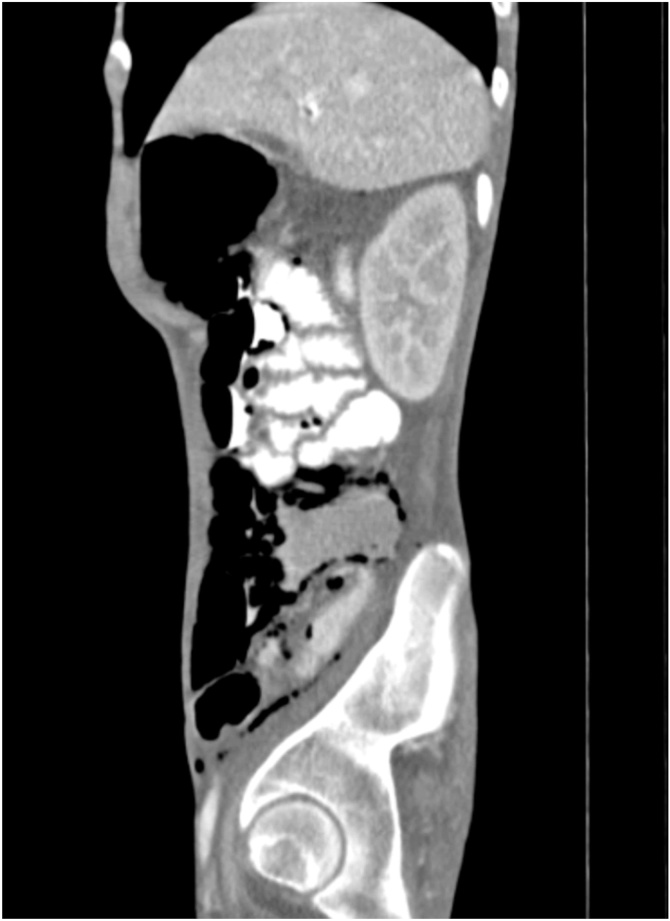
Abdominal sagittal computed tomography image. This abdominal reveals extensive pneumatosis, moderate pneumoperitoneum and gas in the venous portal system.
